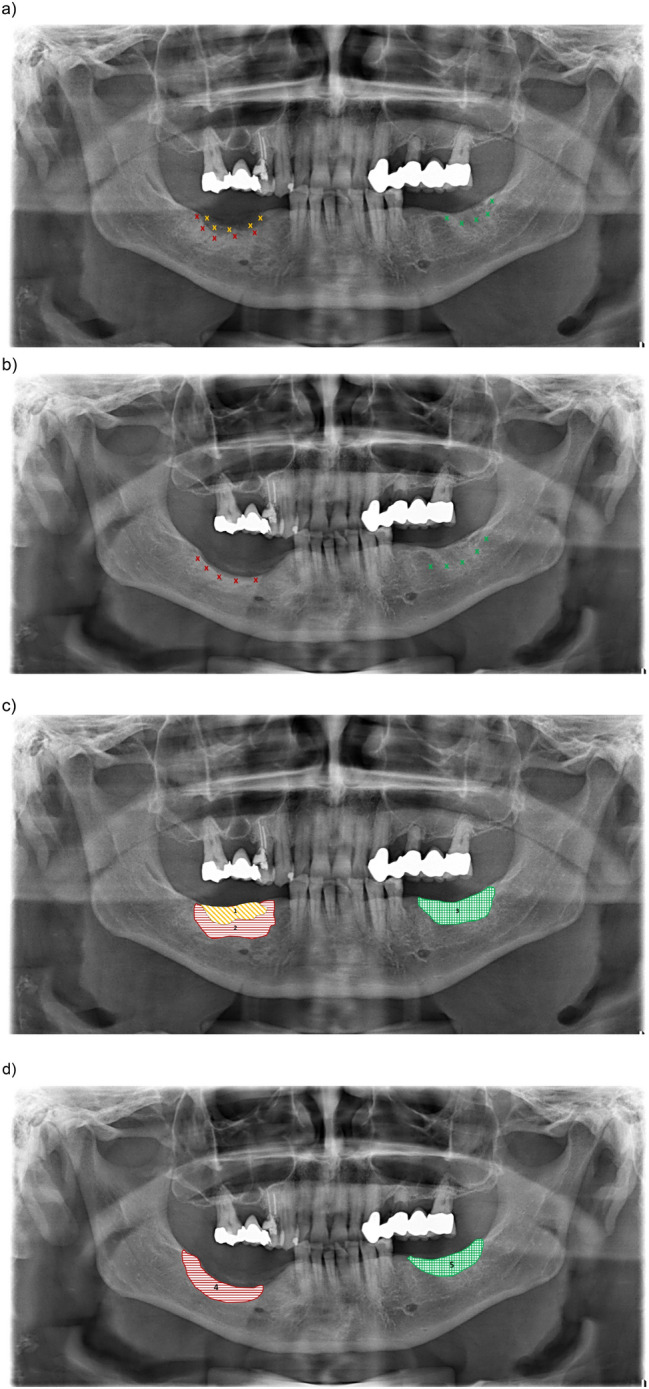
Pre and postoperative panoramic radiograph (a,b) measuring points (Sidexis), (c,d) measured bone zones (ImageJ): 1: osteonecrosis; 2: preoperative sclerotic area; 3: preoperative unaffected reference side; 4: postoperative reactive sclerotic area; 5: postoperative unaffected reference side.
